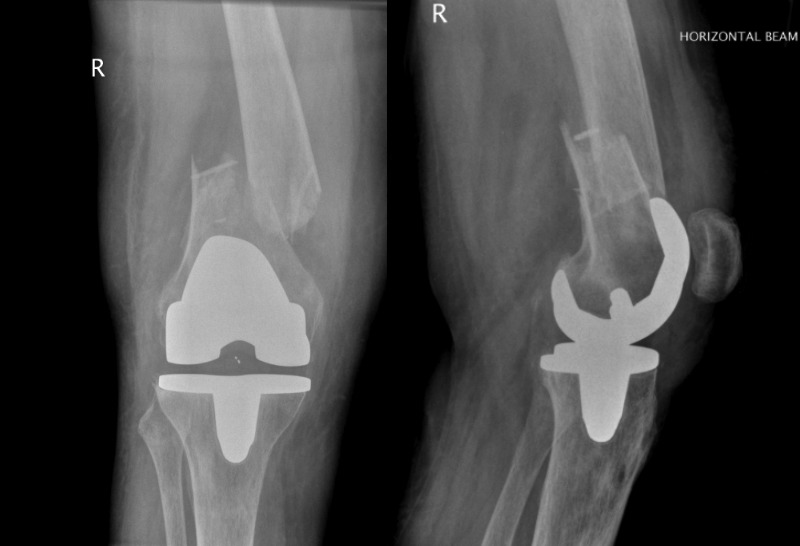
Anteroposterior and lateral radiographs sustaining a Lewis and Rorabeck type II periprosthetic fracture
